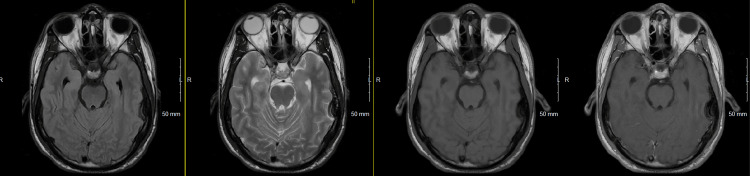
T2 FLAIR, T2 axial, T1, and post-contrast T1 images showing no abnormalities in the bilateral temporal lobes FLAIR: fluid-attenuated inversion recovery
